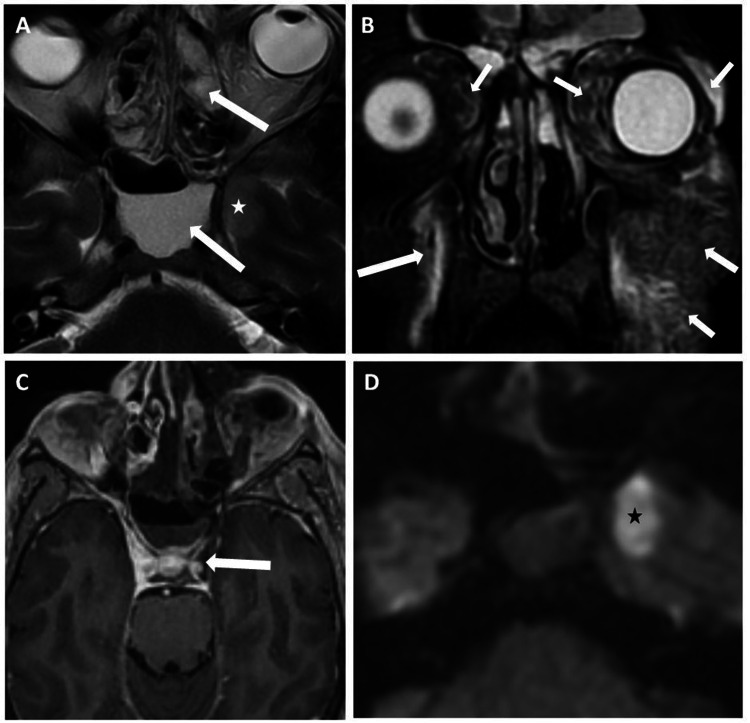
A case of complicated paranasal mucormycosis with extrasinonasal spread to bilateral orbits, para-antral maxillofacial soft tissue, and also left cavernous sinus thrombosis resulting in infarct in the left medial temporal lobe. (A) Axial T2 image showing severe mucosal thickening in bilateral ethmoid sinuses with air-fluid level in the sphenoid sinus (arrows). (Note: A focal hypodensity in the left medial temporal lobe (marked with white star). (B) Altered signal intensity/ edema noted in bilateral extrasinonasal orbital soft tissue and maxillofacial soft tissue (arrows) - suggestive of spread to orbital and maxillofacial soft tissue. (C) Axial T1 post-contrast image showing filling defect in the left cavernous sinus (arrow) causing infarct in the left temporal lobe (white star in A, black star in D). (D) Axial DWI image showing infarct in left temporal lobe (black star). COVID-19: coronavirus disease 2019
